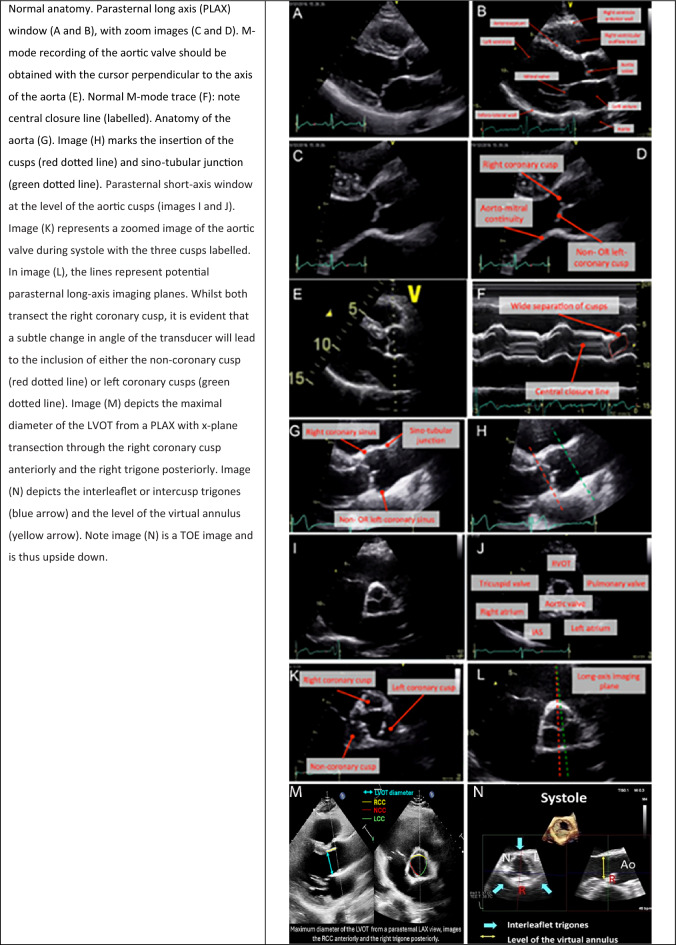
Normal anatomy of the aortic valve and aortic root using echocardiography [13]. Reproduced with permission from Ring et al.
